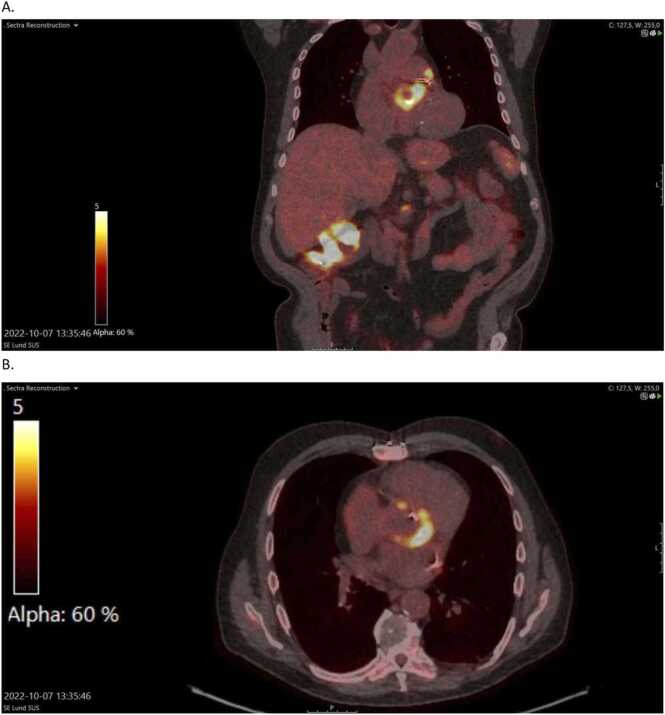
^18^F-fluorodesoxyglucose positron emission tomography–computed tomography (FDG PET-CT) at day 20 of hospitalization. A shows a coronary view and B a transversal view.
